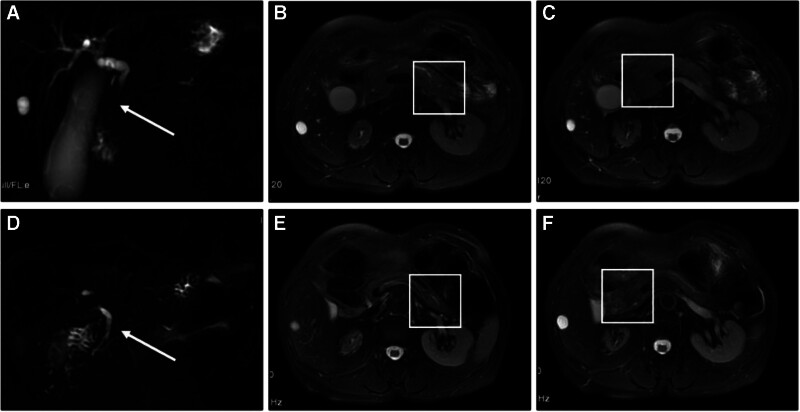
Imaging findings before and after treatment. (A–C) Pretreatment imaging findings. (A) Abdominal MRI showing stenosis of the intrapancreatic common bile duct, and gallbladder enlargement with wall thickening. (B) Abdominal MRI showing enlargement and fullness of the pancreatic body and tail. (C) Abdominal MRI showing enlargement and fullness of the pancreatic head. (D, E) Posttreatment imaging findings. (D) Abdominal MRI showing improvement in the stenosis of the intrapancreatic common bile duct, and a normal-sized gallbladder. (E) Abdominal MRI showing reduction in the fullness of the pancreatic body and tail. (F) Abdominal MRI showing reduction in the fullness of the pancreatic head. MRI = magnetic resonance imaging.
